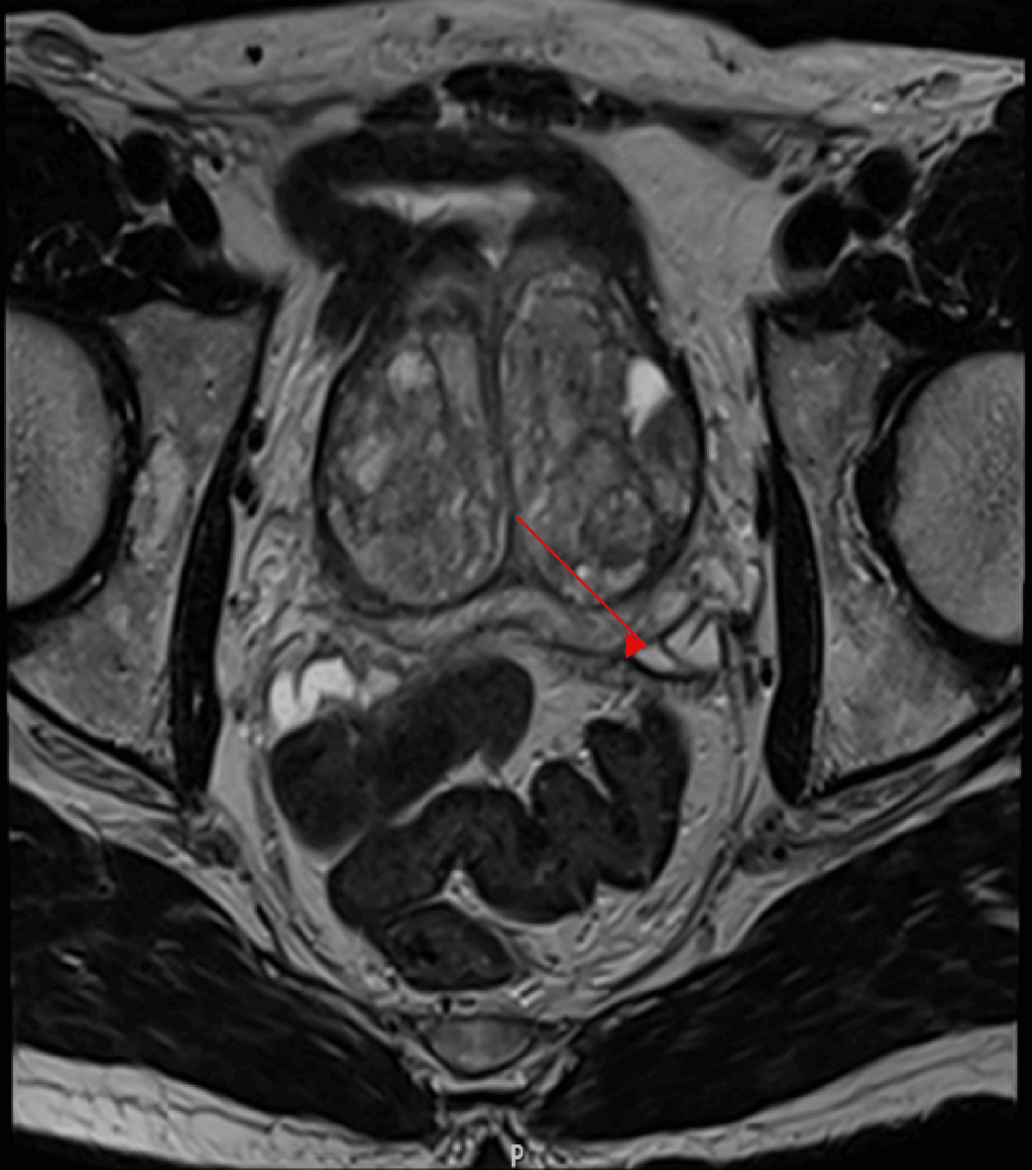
Pre-operative MRI axial view showing enlarged prostate (red arrow)
